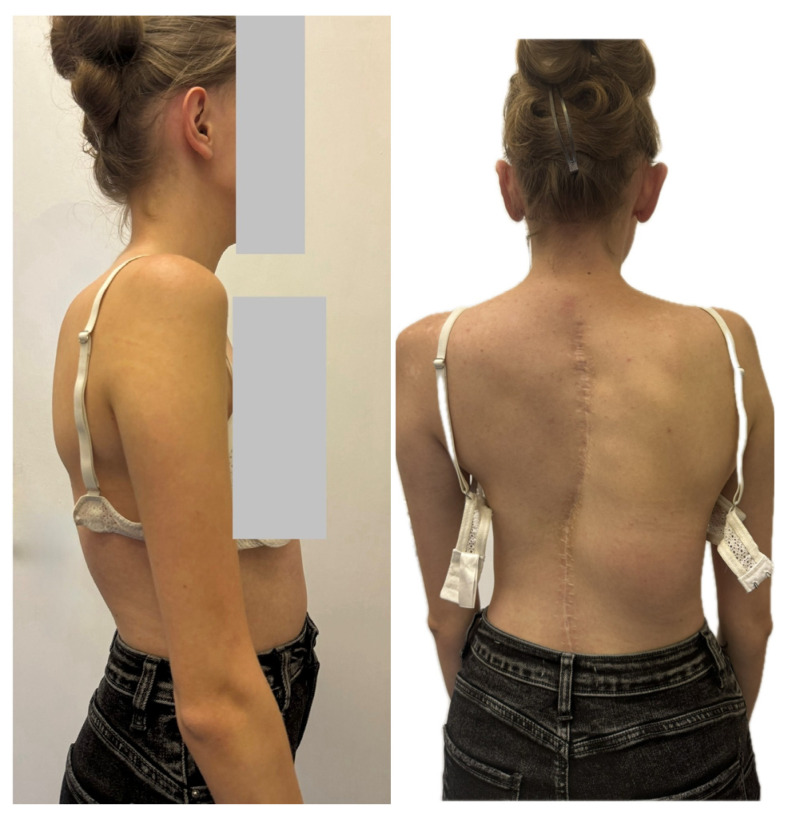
A 17-year-old female patient treated with staged surgery with LITID followed by PSF. Clinical photographs and radiographs performed pre- and postoperatively at final follow-up.
